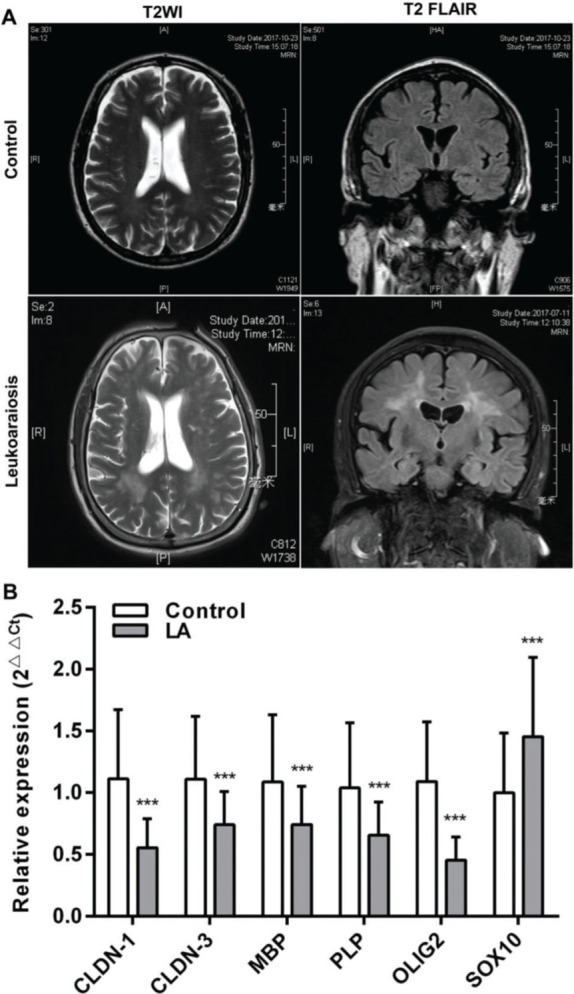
White matter (WM) lesions visualized in normal controls and patients with LA and the relative expression of claudins. (A) The magnetic resonance imaging (MRI) exhibits symmetrical deep lesions located in the periventricular white matter, which showed high signals in T2WI and T2 FLAIR. (B) The expression of claudins (*CLDN-1* and *CLDN-3*) and myelinogenesis-related genes (*MBP*, *PLP*, *OLIG2*, and *SOX10*) in patients with LA. p*<0.001 *versus* the respective control.
